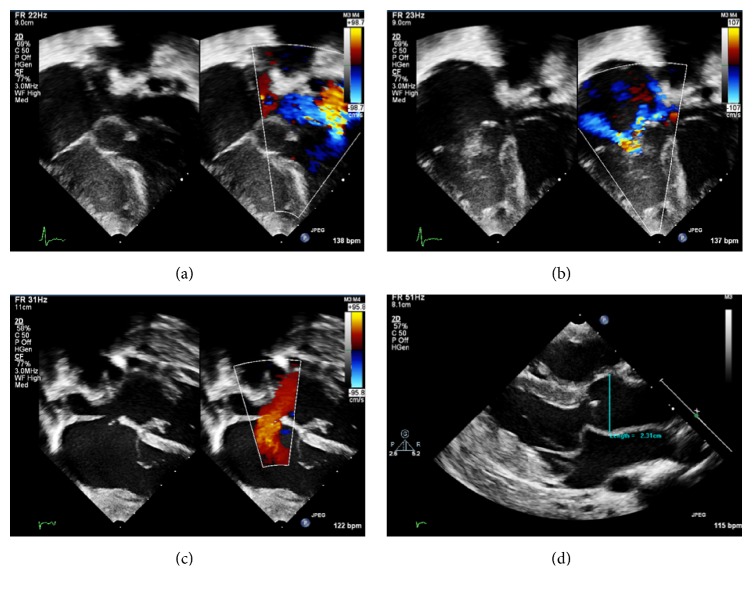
Presurgical echocardiographic features of the proband: (a) apical 4-chamber view demonstrating mitral regurgitation. (b) Apical 4-chamber view demonstrating tricuspid regurgitation. (c) Subcostal 4-chamber view demonstrating atrial septal defect. (d) Parasternal long axis view demonstrating mitral valve prolapse and aortic root dilatation.
